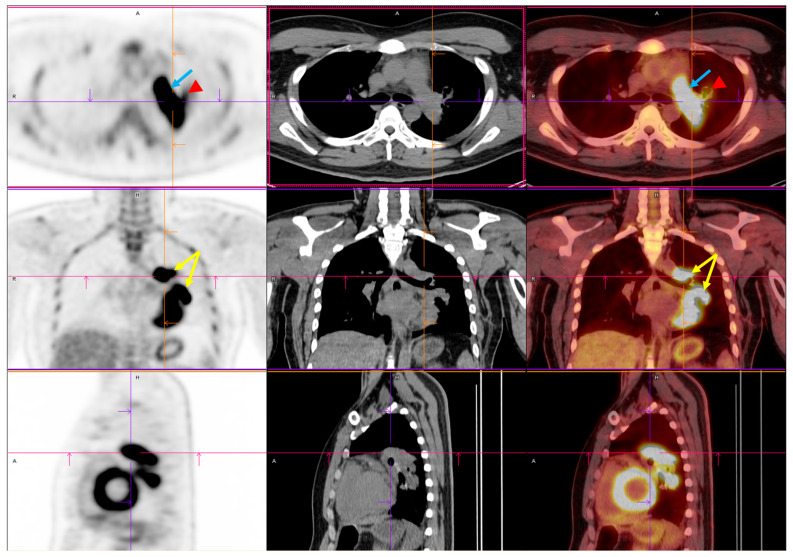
Preoperative PET/CT imaging. FDG PET/CT imaging demonstrated intense FDG uptake involving the left upper and lower lobes within the centrally located mass (yellow arrow) [4,5,6]. Upon initial imaging, the primary lesion exhibited a maximum standardized uptake value (SUVmax) of 14.52, with a delayed SUVmax of 21.08. This indicated significant metabolic activity and a high tumor glycolytic rate. The lesion’s metabolic borders corresponded closely to the soft tissue margins observed on CT, confirming its structural and functional malignancy. Crucially, increased FDG uptake was also identified within the left pulmonary artery (blue arrow) (SUVmax: 12.69), where a lobulated intraluminal filling defect had previously been observed on contrast-enhanced CT. The metabolic activity of the intravascular component mirrored that of the adjacent tumor, further supporting the diagnosis of a tumor thrombus rather than a bland thromboembolism [7]. This pattern of contiguous metabolic extension from the primary lung mass into the pulmonary vasculature strongly suggested direct vascular invasion. Mildly increased uptake was noted in the left hilar region (red arrowhead). This raised concerns about potential nodal involvement. Abbreviations: FDG, 18F-fluorodeoxyglucose; PET/CT, positron emission tomography/computed tomography; SUVmax, maximum standardized uptake value.
